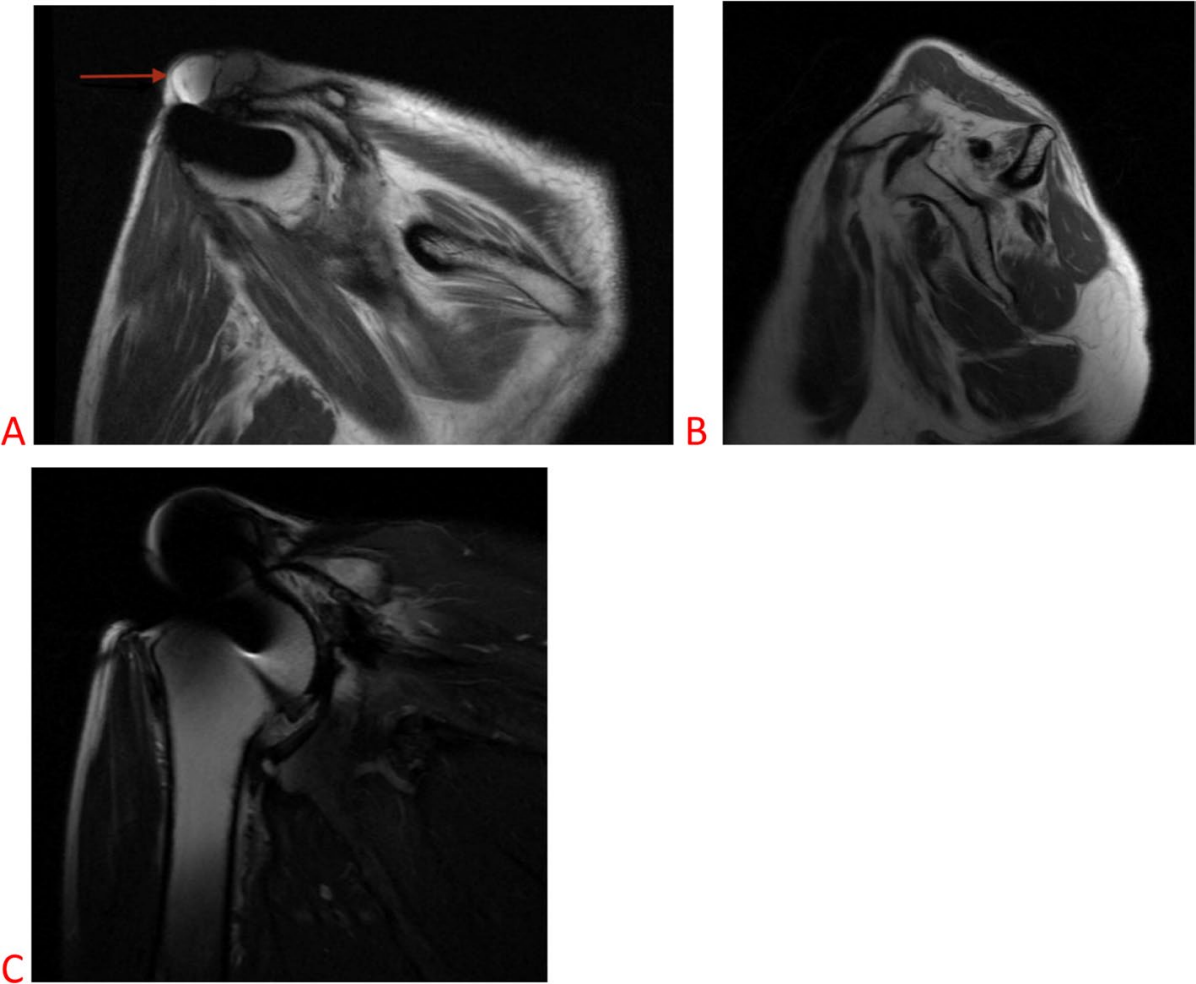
MRI of the right shoulder. A Coronal proton density image showing a cystic mass overlying the acromion (straight arrow); B Sagittal T1 MRI demonstrating severe fatty infiltration of the supraspinatus and infraspinatus muscles; and (C) Coronal T1 MRI demonstrating a massive rotator cuff tear with retraction of the superior rotator cuff to the level of the glenoid
